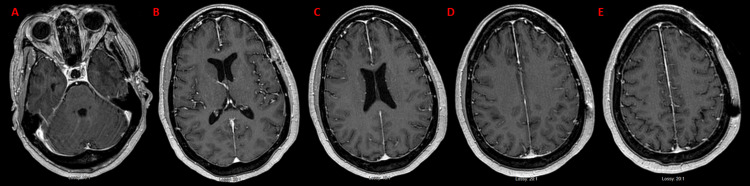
MRI brain after completion of four cycles of D-RVd. MRI post-T1 axial series show (A-E) no intra- or extraaxial masses are found. D-RVd: daratumumab, lenalidomide, bortezomib, dexamethasone
